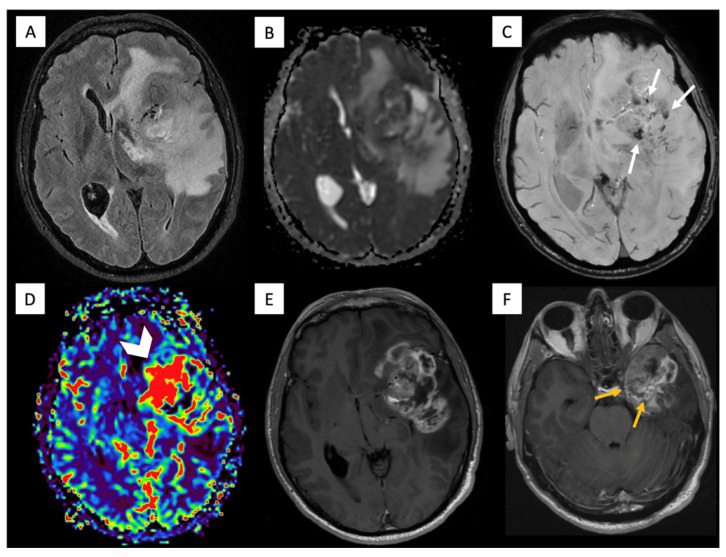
Brain MRI of Patient 2. Brain MRI axial FLAIR (A), ADC (B), T2 star-weighted angiography (SWAN) (C), DSC perfusion (D), and contrast-enhanced T1-weighted (E,F) images show a frontotemporal lobe mass with a necrotic center and peripheral enhancement, edema, high-grade ITSS (white arrows), and increased rCBV (arrowhead). Dural extension and ependymal infiltration of the temporal horn are also noted (yellow arrows).
